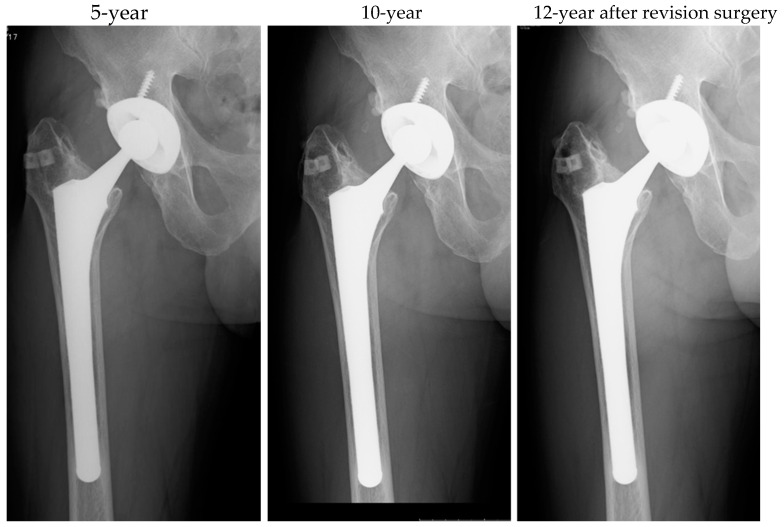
Anteroposterior radiograph after revision surgery in case 9.
